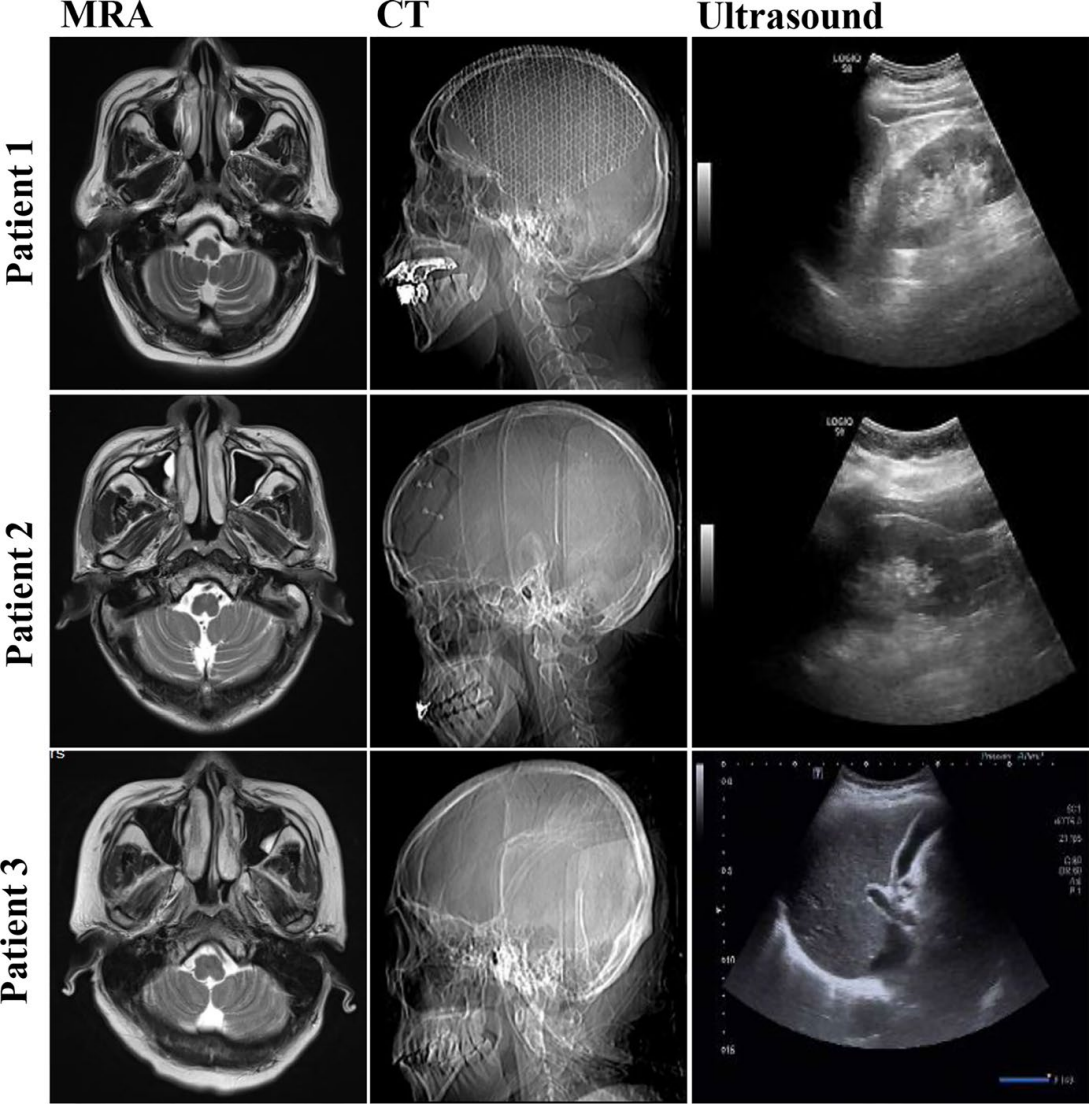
Preliminary medical imagines of valproic acid (VPA)‐treated epileptic patients. As shown in diagnostic images of magnetic resonance angiography (MRA) and computed tomography (CT), the VPA‐treated epileptics resulted in the brain impairment, mild leukoaraiosis, and brain atrophy. However, normal morphology and structure of other key internal organs of liver, spleen, kidney were detected in ultrasonic examination
